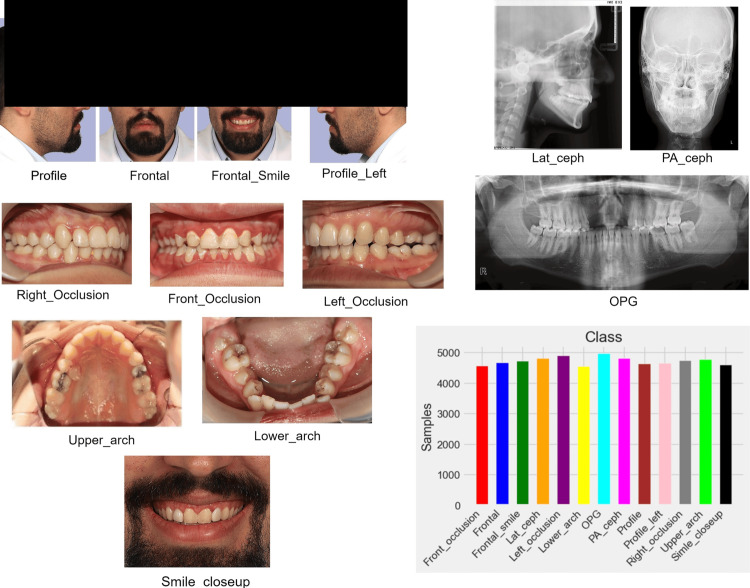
Orthodontic diagnostic records and the quantity of each type included in this study. Lat-Ceph: lateral cephalometry; OPG: orthopantomograph; PA-Ceph: posteroanterior cephalometry
